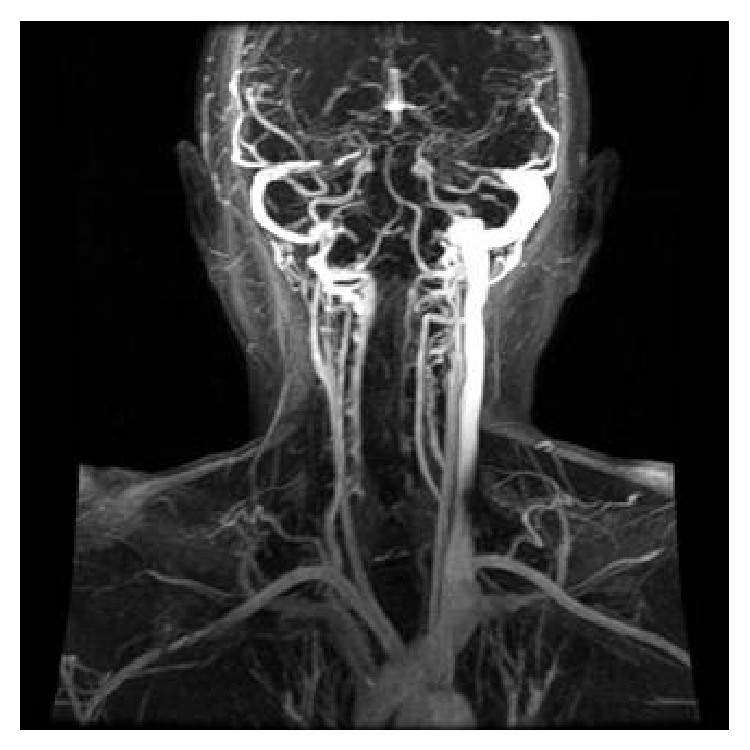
Sagittal view of venous system (dynamic magnetic resonance image). Please note that right filling of IJV with the radiopaque contrast is absent, markedly, from the bifurcation. Moreover, left IJV is seen thickened.
